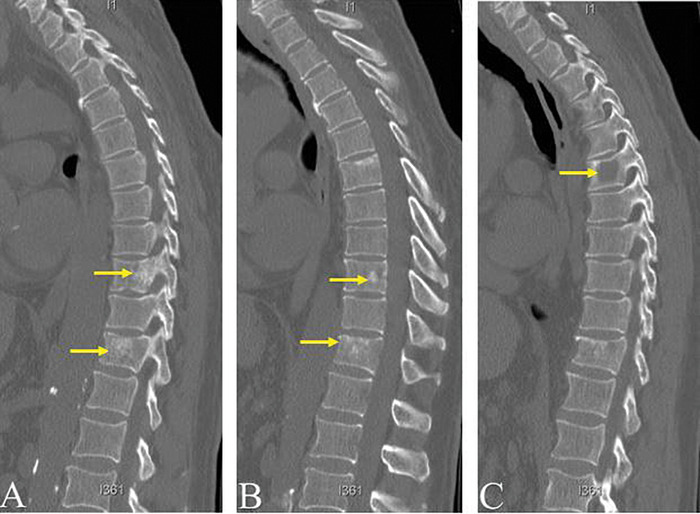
Sagittal computed tomography sections without contrast of the thoracic spine demonstrate multiple mixed sclerotic/lytic lesions involving the T9 and T11 vertebral bodies (A and B), with a lytic lesion at T6 (C). Lumbar lesions are not shown.
